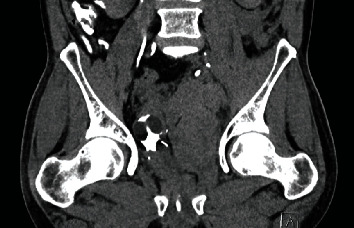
CT pelvis with cystogram at our hospital (coronal): displaced bladder; poor cystogram without extravasation.
